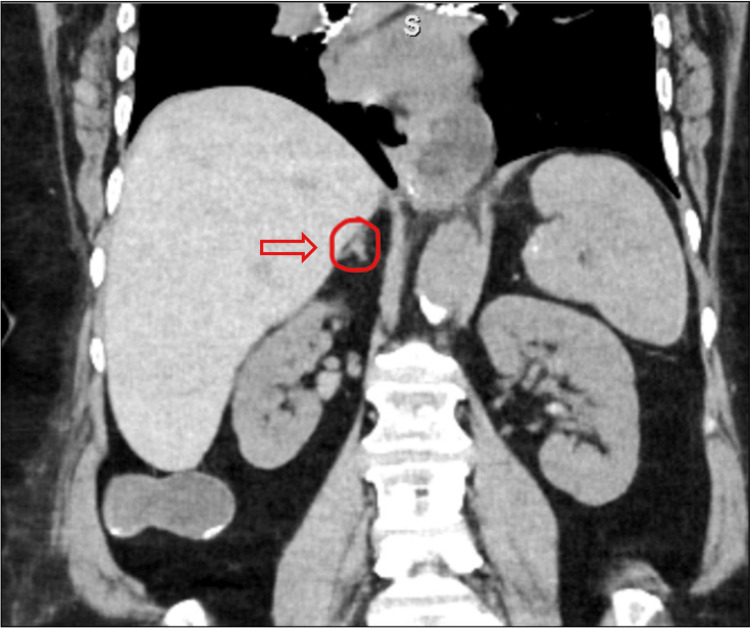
Abdominal CT showing atrophy of adrenal glands
